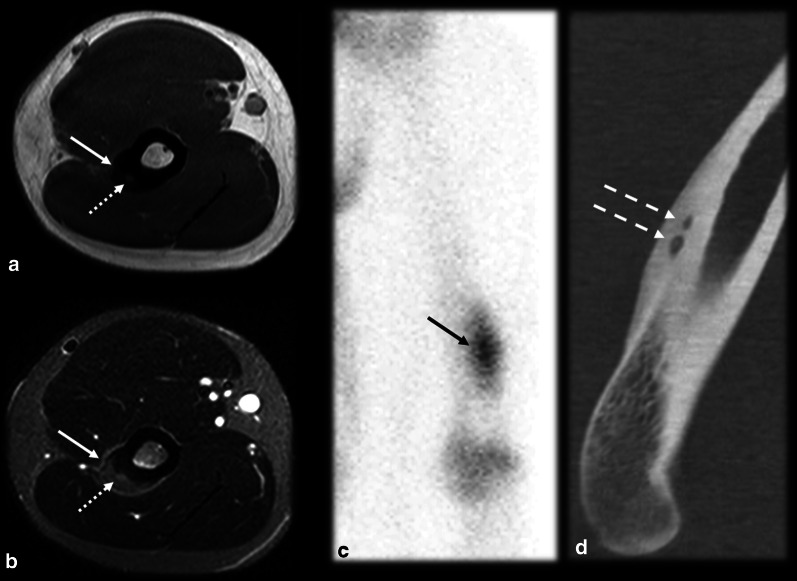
Multicentric osteoid osteoma. A 26-year-old male handball player with lateral elbow and arm pain for 3 months. Orthopedists suspected lateral epicondylitis or a stress reaction. MRI T1 (a) and T2 FS (b) showed cortical thickening on the lateral supracondylar crest with corticoperiosteal edema (arrow) and small foci of intermediate signal intensity (dotted arrow), which raised the suspicion for OO. Scintigraphy (c) evidenced the double density sign (black arrow), which cannot be used to distinguish between single and multicentric OO. CT (d) detected two nidi (dashed arrows), confirming the diagnosis of multicentric OO
